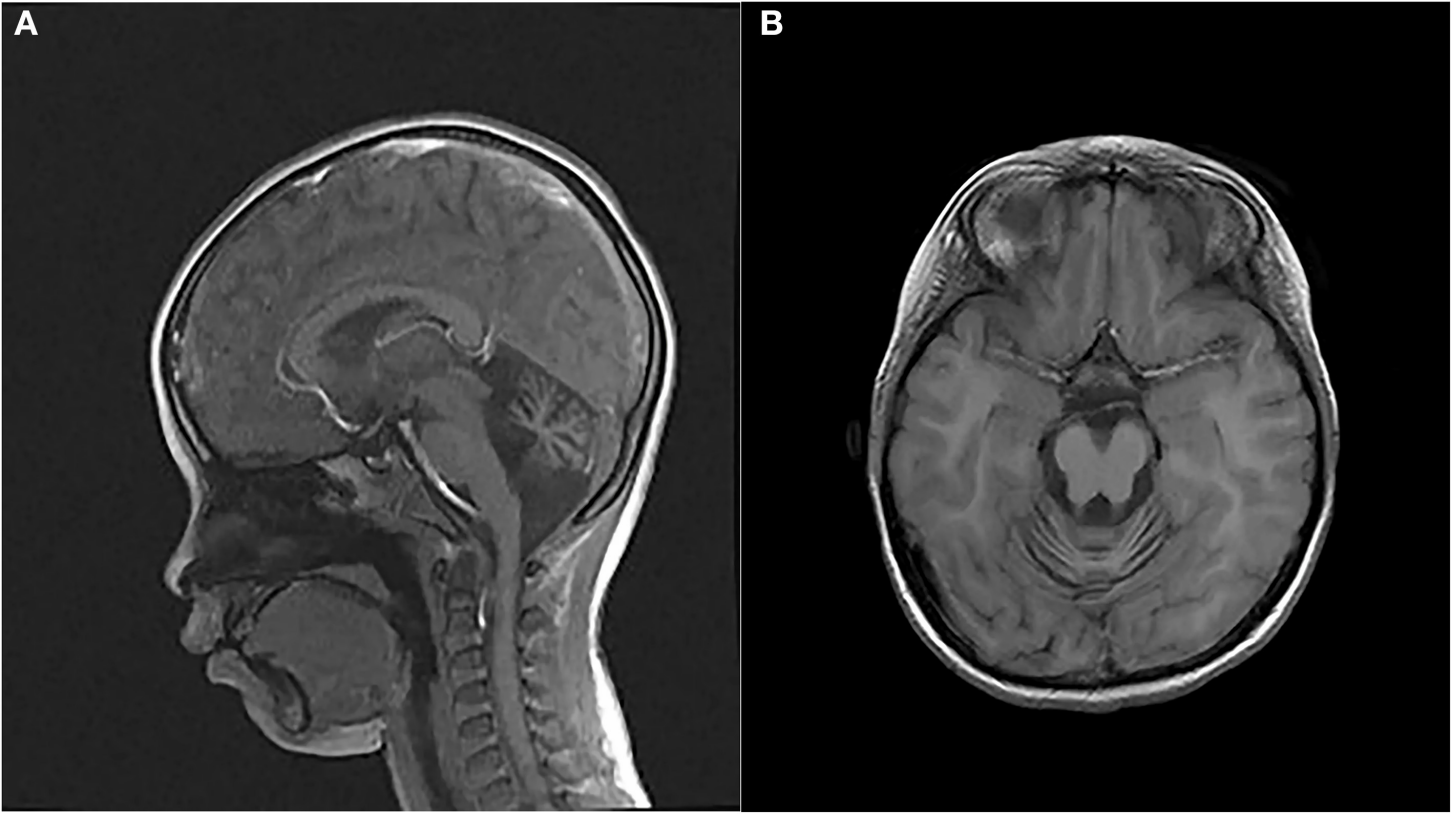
Magnetic resonance images (MRIs) of the patient's brain. (A) Sagittal T2-weighted brain MRI. (B) Axial T1-weighted brain MRI.
